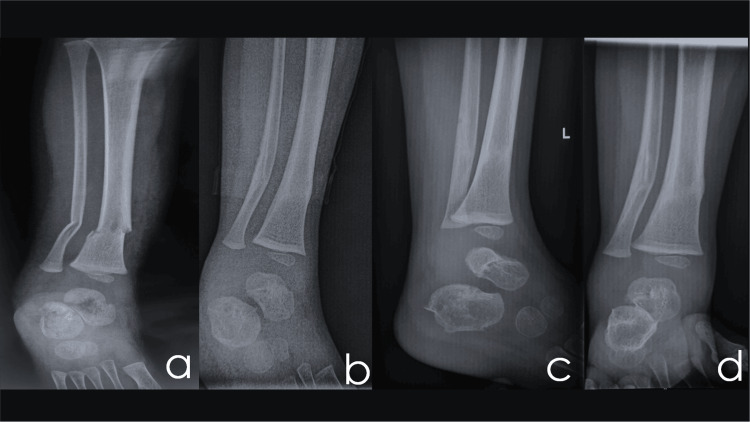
Radiographic course during follow-ups (a) X-ray (face) at the diagnosis, (b) X-ray (face) at one week, (c) X-ray (profile) at three weeks, and (d) X-ray (face) at three weeks
